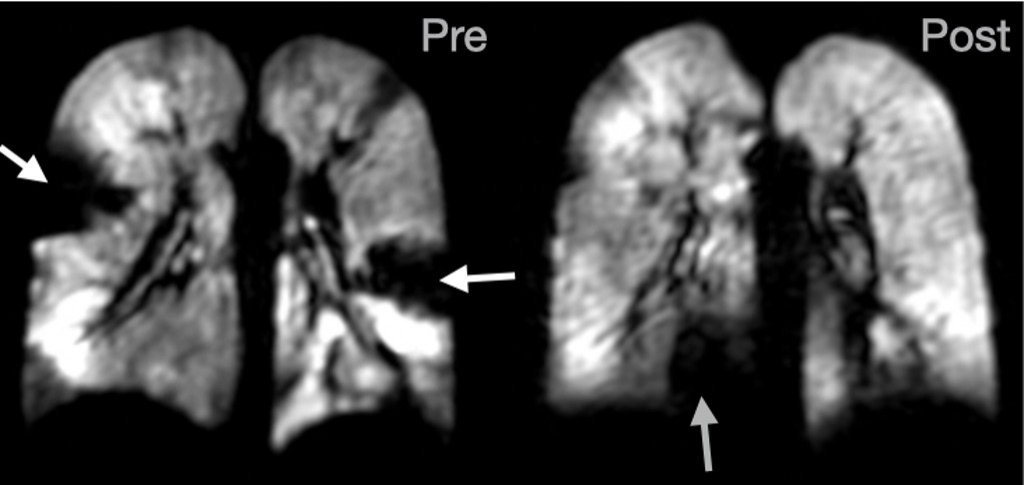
Patient D: clinical diagnosis of non-CF bronchiectasis. The patient suffered from a chronic productive cough and amongst other treatments had a two-week course of IV antibiotics three months prior to referral for MRI. The patient’s FEV_1_ was static at >90%-predicted and historically did not change after antibiotics, making it difficult to determine the treatment efficacy. The patient was therefore referred for pre- and post-therapy ventilation MRI during their next two-week course of IV antibiotics. Large ventilation defects were evident in both lungs in baseline ventilation images (FEV_1_ = 94%). Post-therapy, the patient’s FEV_1_ decreased to 89%-predicted, whilst ventilation images exhibited almost complete resolution of the large ventilation defects that were present at baseline (white arrows), although a new ventilation defect was present in the basal right lung (grey arrow). These images helped reassure the clinical team as to the efficacy of the current treatment regime. This case highlights the sensitivity and benefit of regional lung function assessment offered by HP gas MRI in children on therapy, and the relative insensitivity of FEV_1_ to detect these changes. CF, cystic fibrosis;
